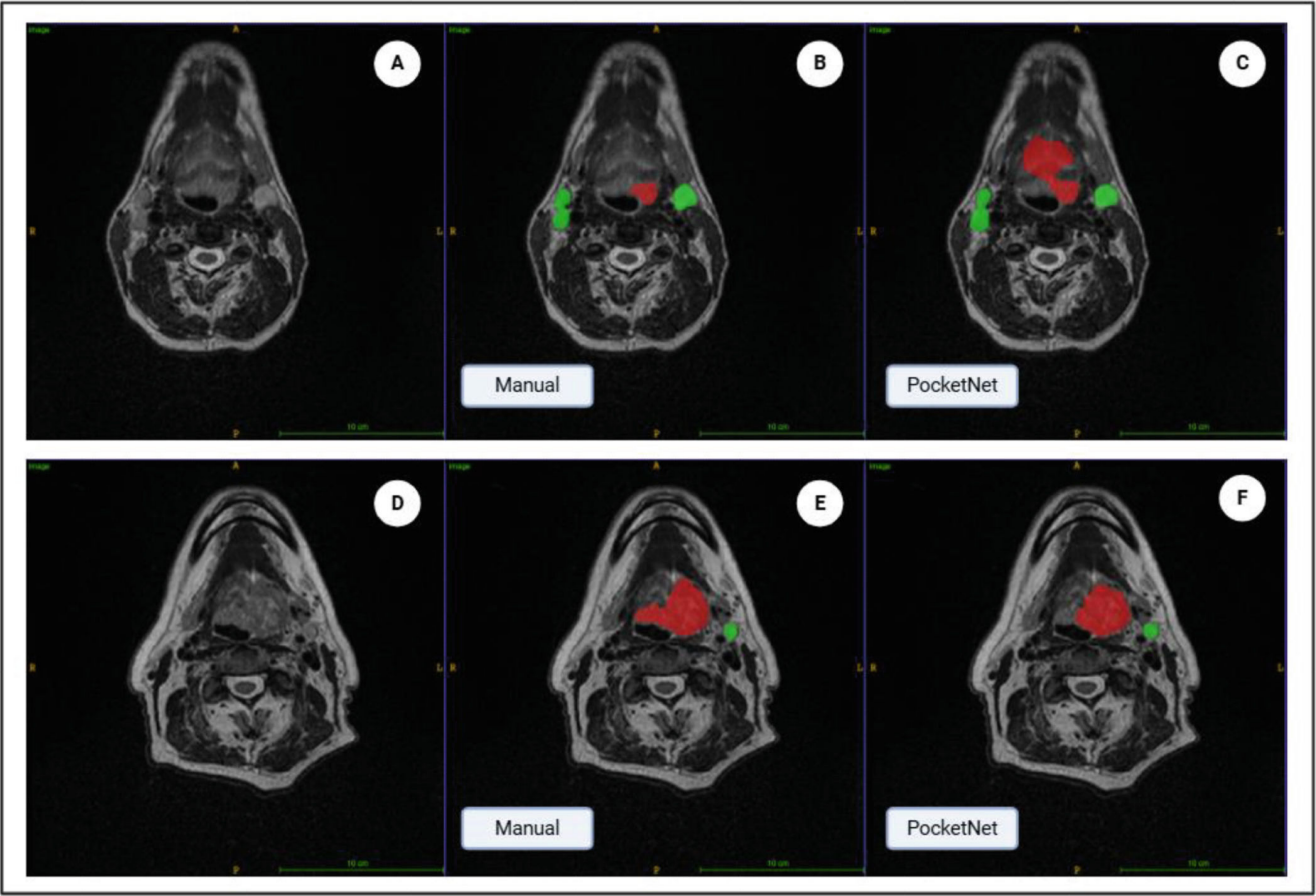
Visual results of segmentation in two patients. A) Axial MRI of Case 3. B) Case 3 manual segmentation of GTVp (red) and GTVn (green). C) Case 3 automated seg-mentation of GTVp (red) and GTVn (green). D) Axial MRI of Case 4. E) Case 4 manual segmentation of GTVp (red) and GTVn (green). F) Case 4 automated segmentation of GTVp (red) and GTVn (green). The segmentations demonstrate poor agreement of gross tumor volumes with a DSC of 0.51 for GTVp and 0.78 for GTVn for Case 3 and 0.75 DSC for GTVp and 0.58 for GTVn for Case 4.\CFO{}
